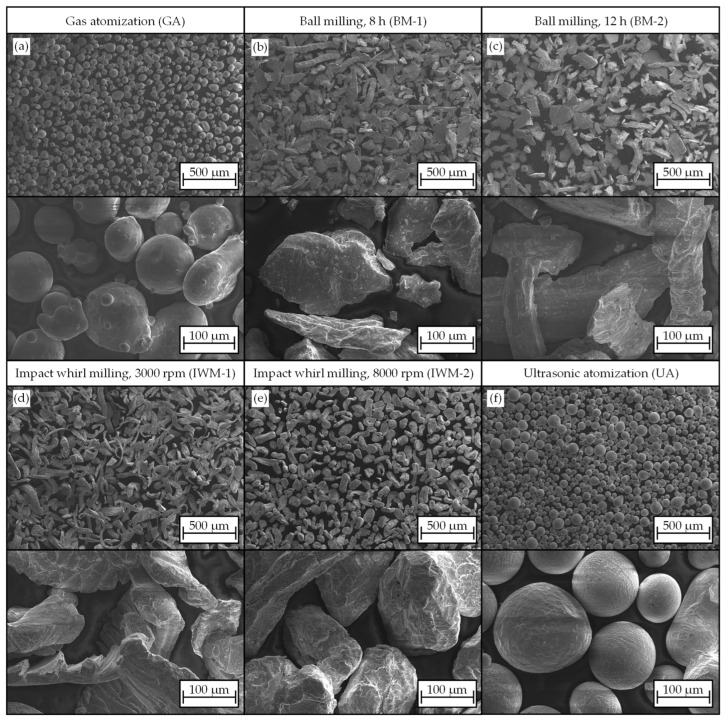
Overview of SEM images showing the materials of this study: Gas atomized reference powder (a), ball milled chips after t_mill_ = 8 h (b) and t_mill_ = 12 h (c), chips from the impact whirl mill at n_mill_ = 3000 rpm (d) and n_mill_ = 8000 rpm (e), powder produced via ultrasonic atomization (f).
